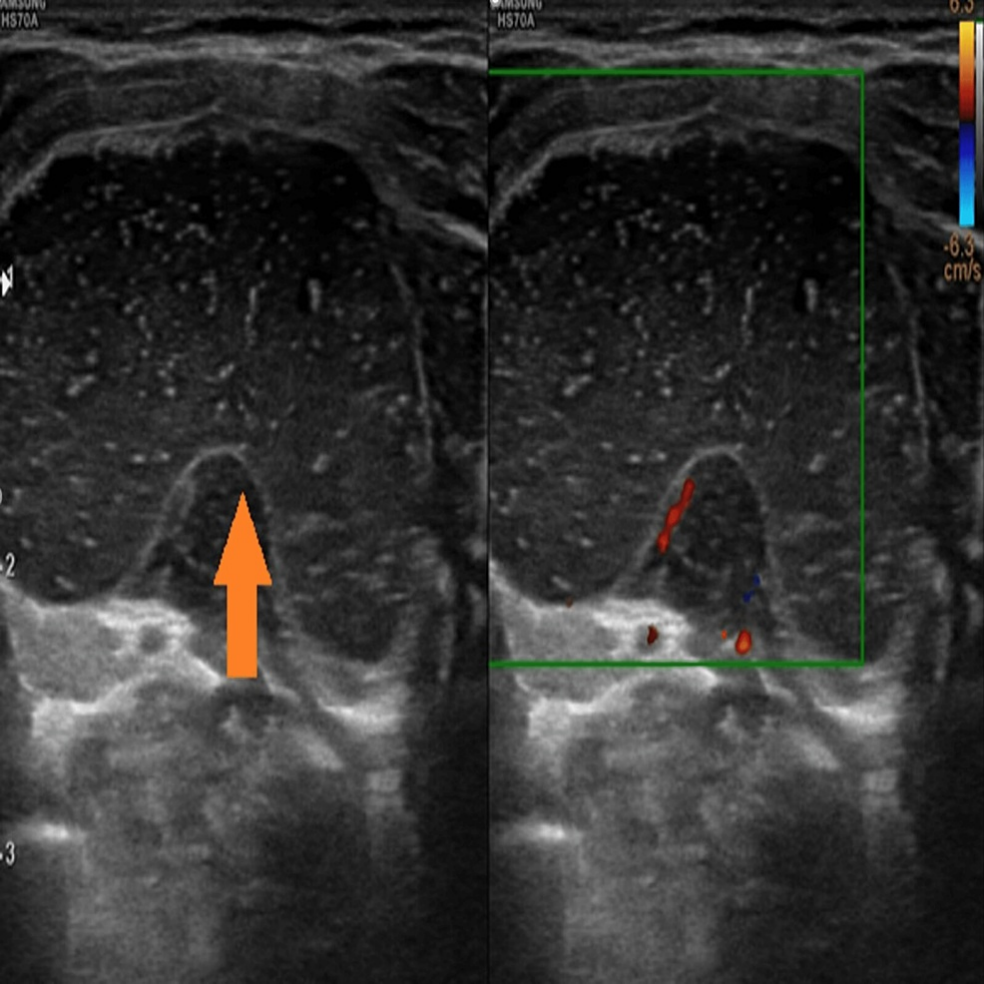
B-mode ultrasound of the submandibular region showing the submandibular component of the ranula (orange arrow) as a morphologically similar well-defined cystic lesion with multiple moving internal echoes within it. No vascularity was demonstrated on color Doppler. No calcification or septation was seen.
